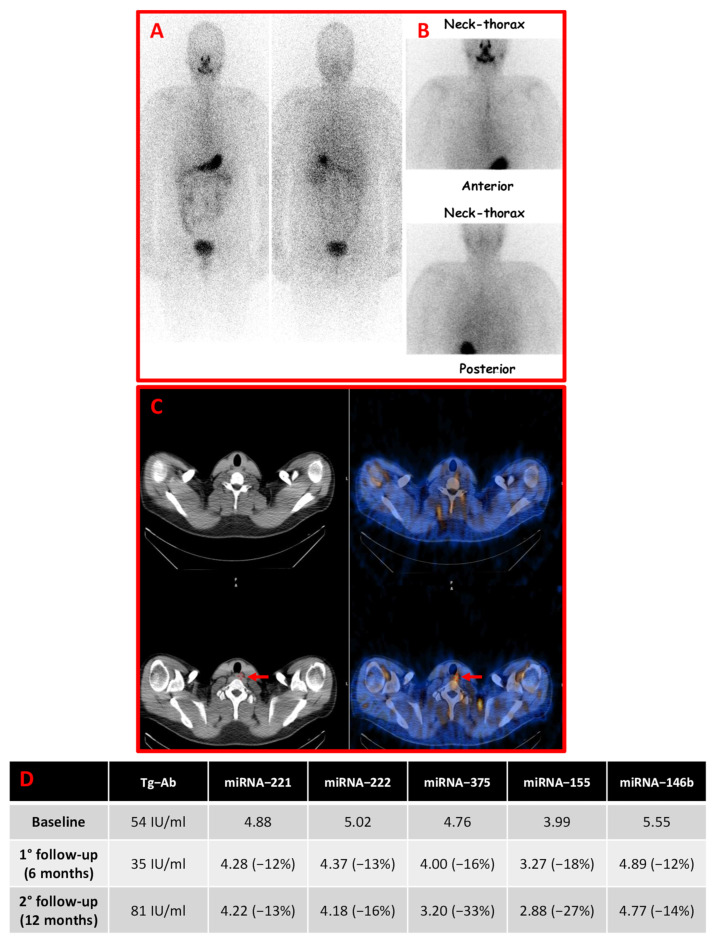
A male PTC patient, aged 54 years, with a multifocal and bilateral PTC [pT1b(m), Nx, Mx], and incomplete structural response (SIR) after initial treatments (surgery + RIT). Imaging findings and serum miRNAs changes are reported. Panel (A,B) ^123^I-Dx-WBS (222 MBq; anterior and posterior views) and planar imaging of the neck-thoracic region (anterior and posterior views). No abnormal radioiodine uptake was noted. Panel (C) SPECT/CT imaging (axial images) showed an abnormal radioiodine uptake involving at least a small-sized lymph node of the VI Robbins’ level (red arrows). (D) Changes in TgAb and circulating miRNAs 221, 222, and 146b levels during the follow-up in the patient.
